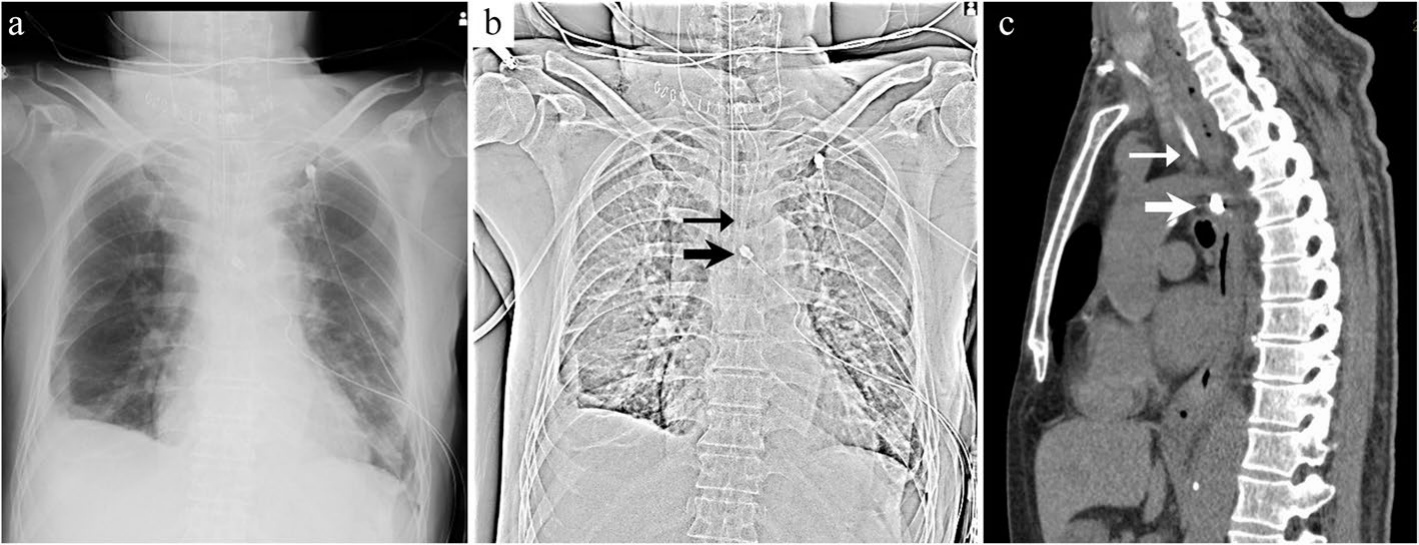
a, b Postoperative chest radiography image. c Postoperative chest computed tomography (CT) image. The tips of the mediastinal drain and chest drain are in close proximity. The thin arrow indicates the tips of the mediastinal drain. The thick arrow indicates the tips of the drain on the dorsal side of the esophagus through the chest cavity
